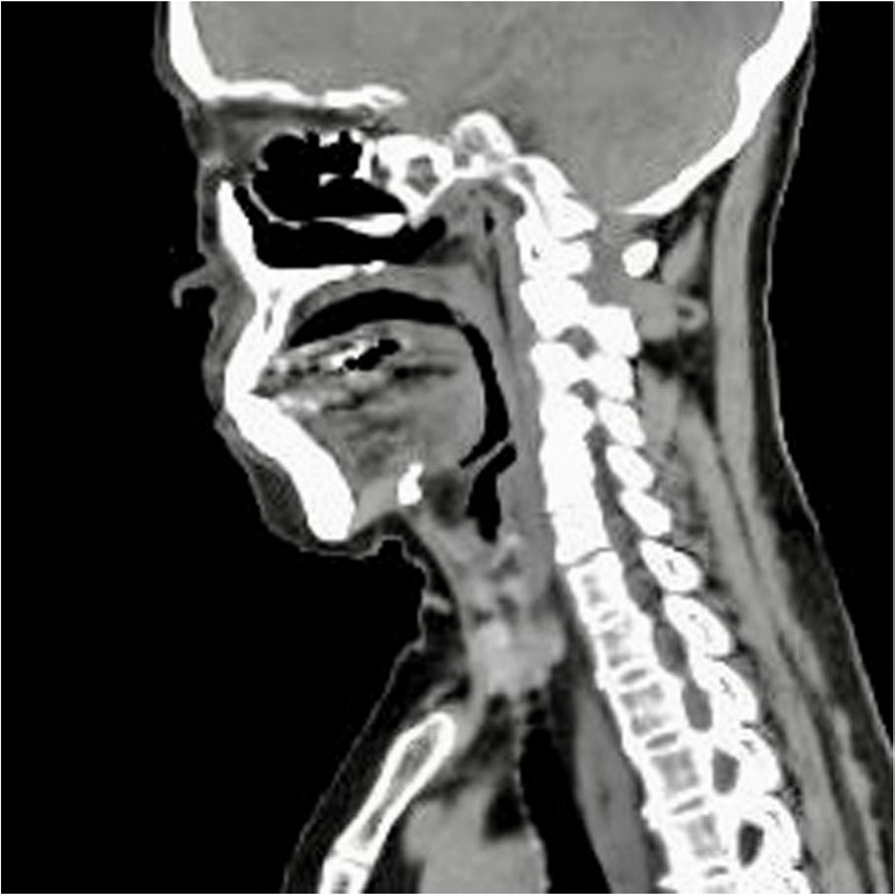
Sagittal computed tomographic view of the upper airway
